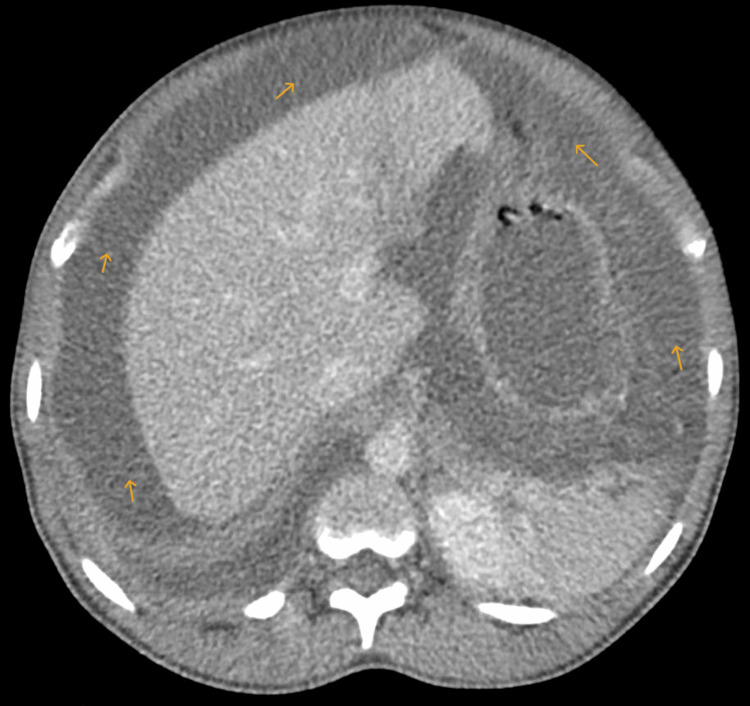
CT scan showing accumulation of ascitic fluid in the peritoneal cavity (yellow arrows) CT: computed tomography
